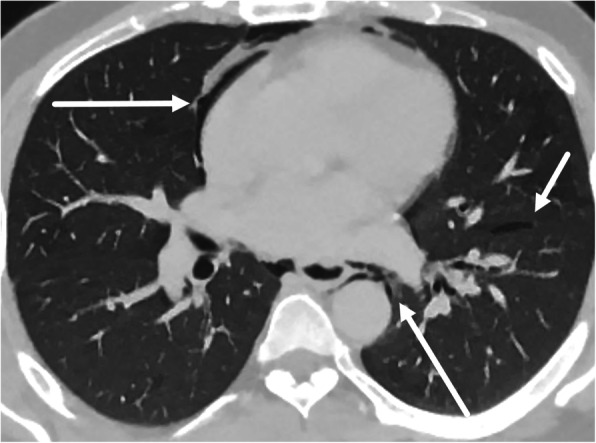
Pneumomediastinum. Thirty-four-year-old male with chest pain after vigorous cough. Axial CT image shows moderate pneumomediastinum (long arrow) caused by Macklin effect. Subpleural gas (short arrow) caused by alveolar rupture in the setting of increased intrathoracic pressure with air dissecting along the subpleural interstitium, interlobular septa, peribronchovascular interstitial sheaths, and eventually into the mediastinum (Macklin effect). While this mimics EP, there is a lack of inflammatory changes and hydropneumothorax commonly associated with esophageal perforation
